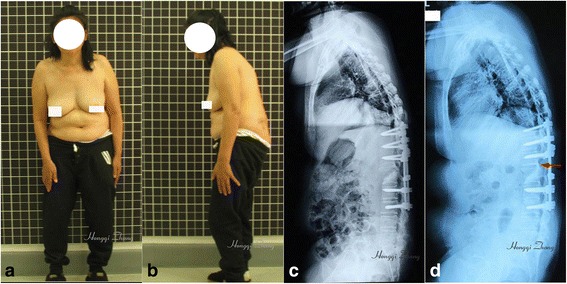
Result of osteotomy through the fracture gap in the female patient with ankylosing spondylitis. a, b Photographs show satisfactory correction achieved via osteotomy through the pathological fracture gap. c Radiograph shows stable internal fixation (without displacement) and corrected kyphosis. d Radiograph at follow-up after 1 year shows the closed fracture gap and stable bone fusion achieved at the posterior column
